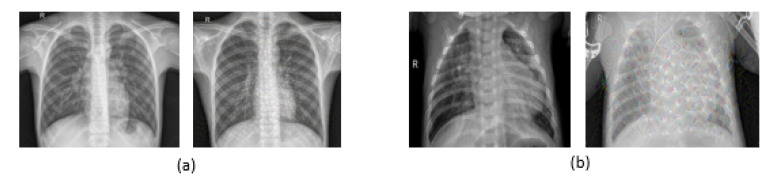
Chest X-ray images: (a) Normal images with and without a deep dream filter; (b) pneumonia images with and without a deep dream filter.
